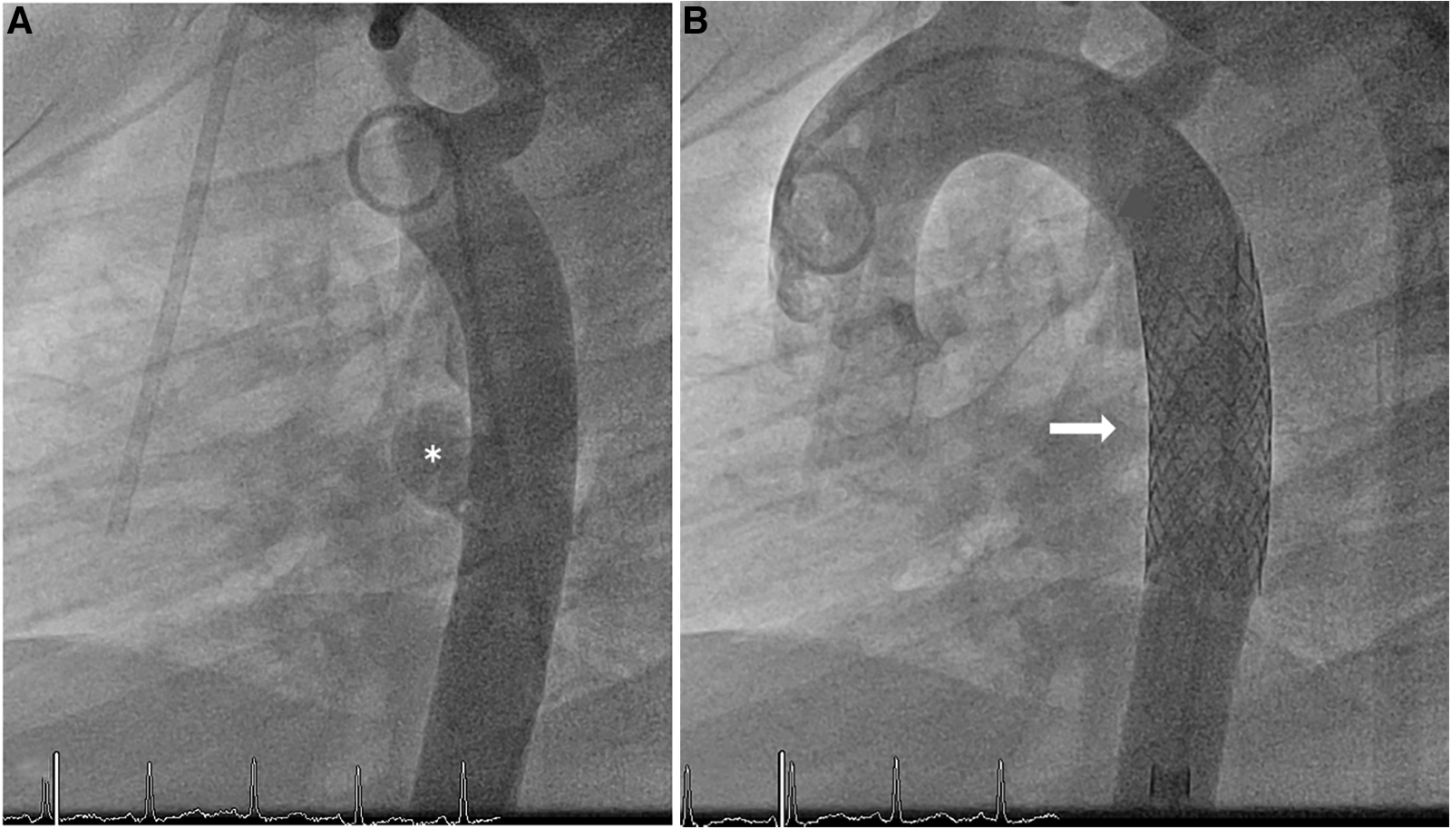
Thoracic CT scan (A) pre-intervention, (B) post-intervention. * indicates a mycotic aneurysm in the wall of the descending aorta;
